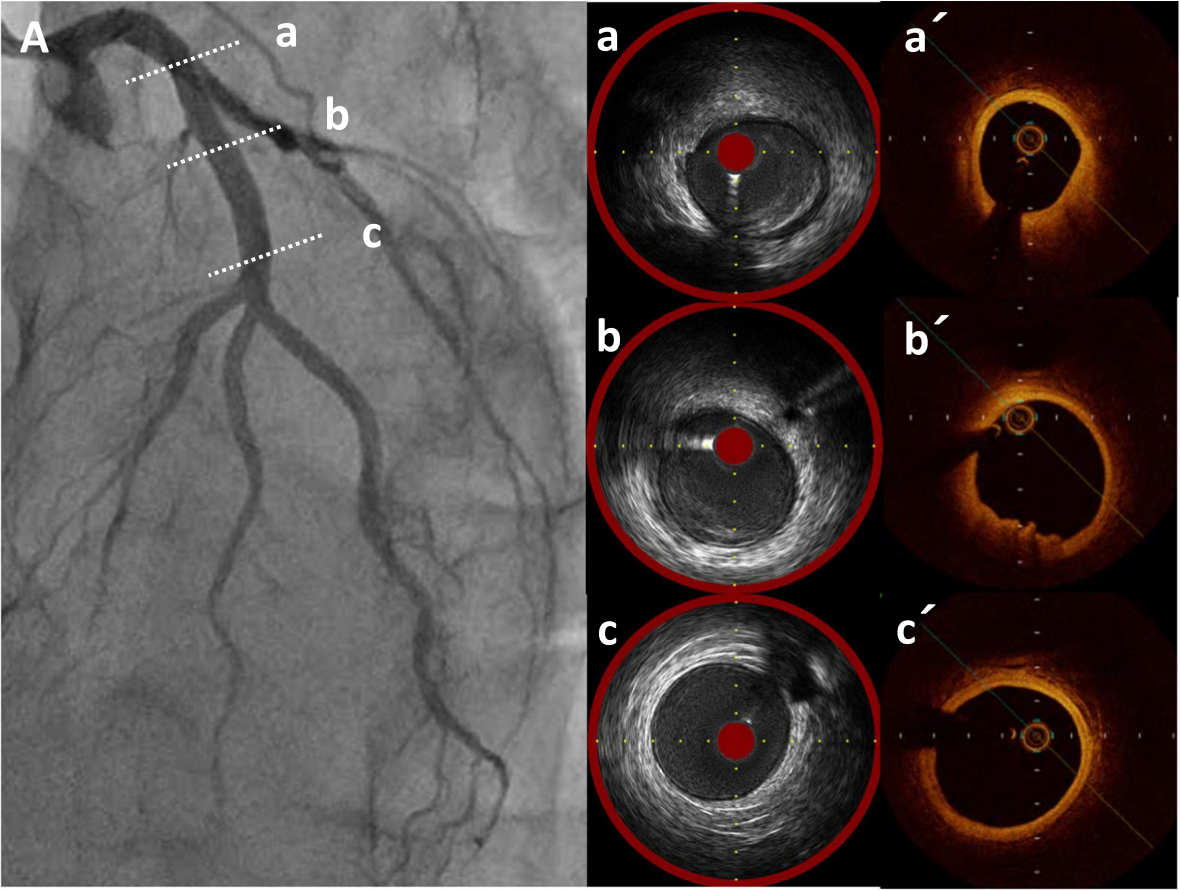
The findings of near-infrared spectroscopy–intravascular ultrasonography and optical coherence tomography in 1 week. Coronary angiography showed further reduced thrombus without severe stenosis in the left anterior descending (LAD) artery. The findings of near-infrared spectroscopy–intravascular ultrasonography (A-a, b, c) and optical coherence tomography (A-a´, b´, c´) revealed a small amount of residual thrombus in the short segment and mild fibrous plaque in the proximal left anterior descending artery
